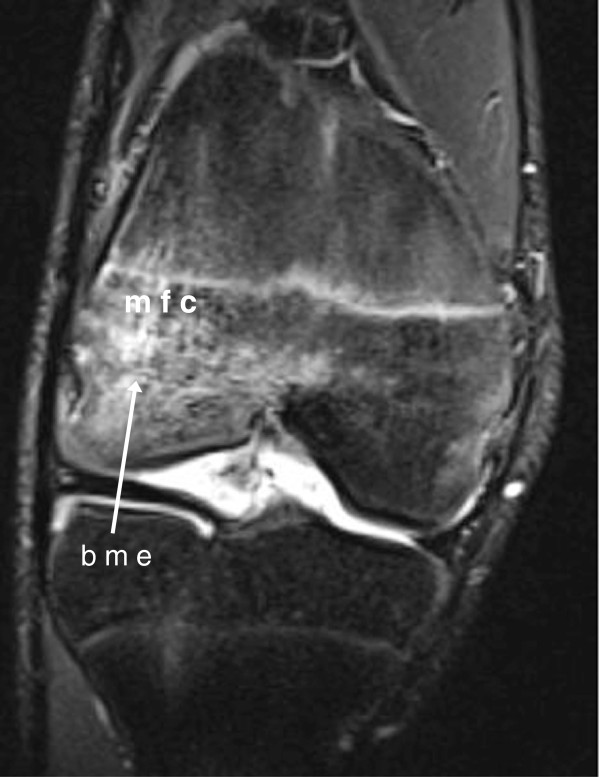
Imaging of the symptomatic knee of a 14-year-old boy with JIA. Coronal STIR MRI image showing bone marrow edema (bme) in the medial femoral condyle (mfc).
